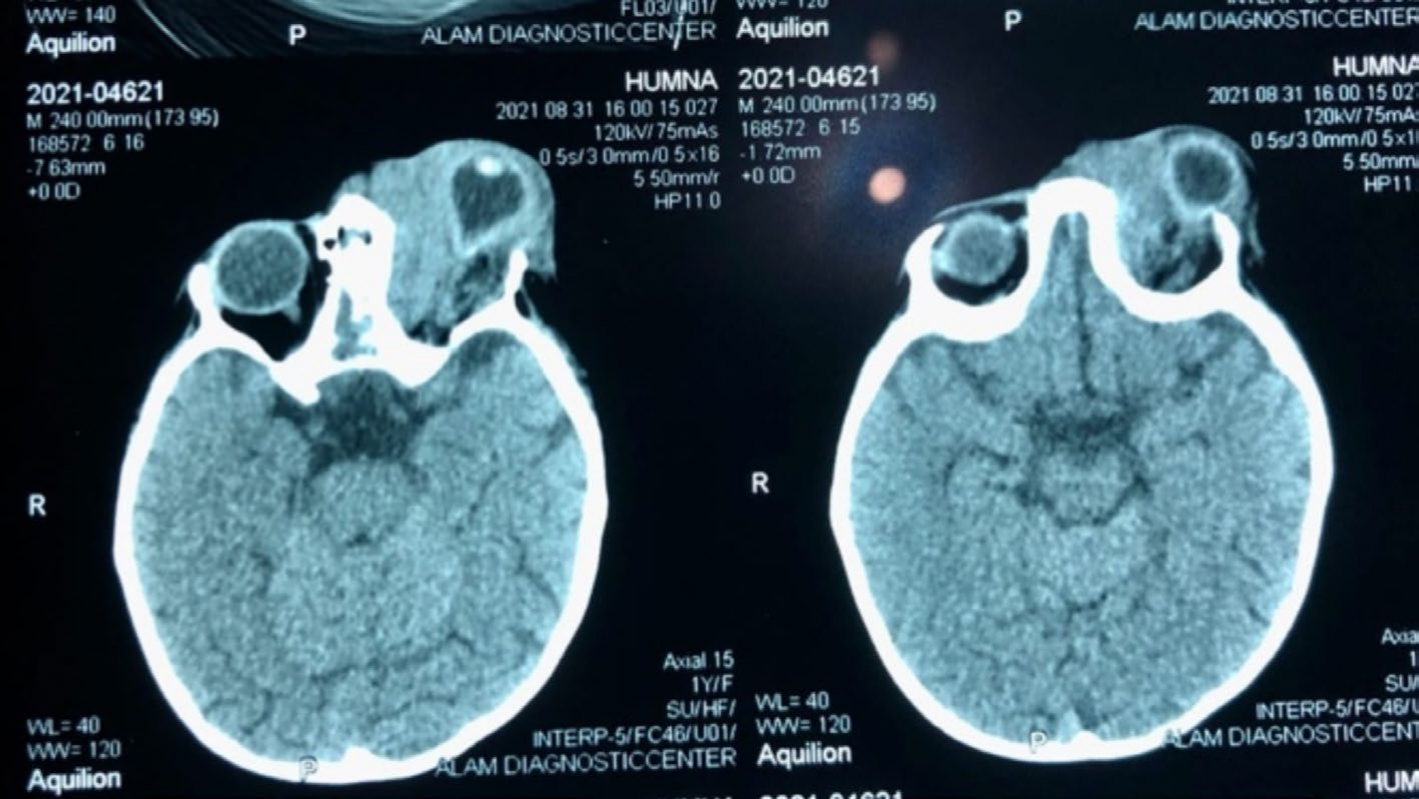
CT scan showing sinusitis involving the right ethmoid and maxillary sinuses, along with the orbital mass.
